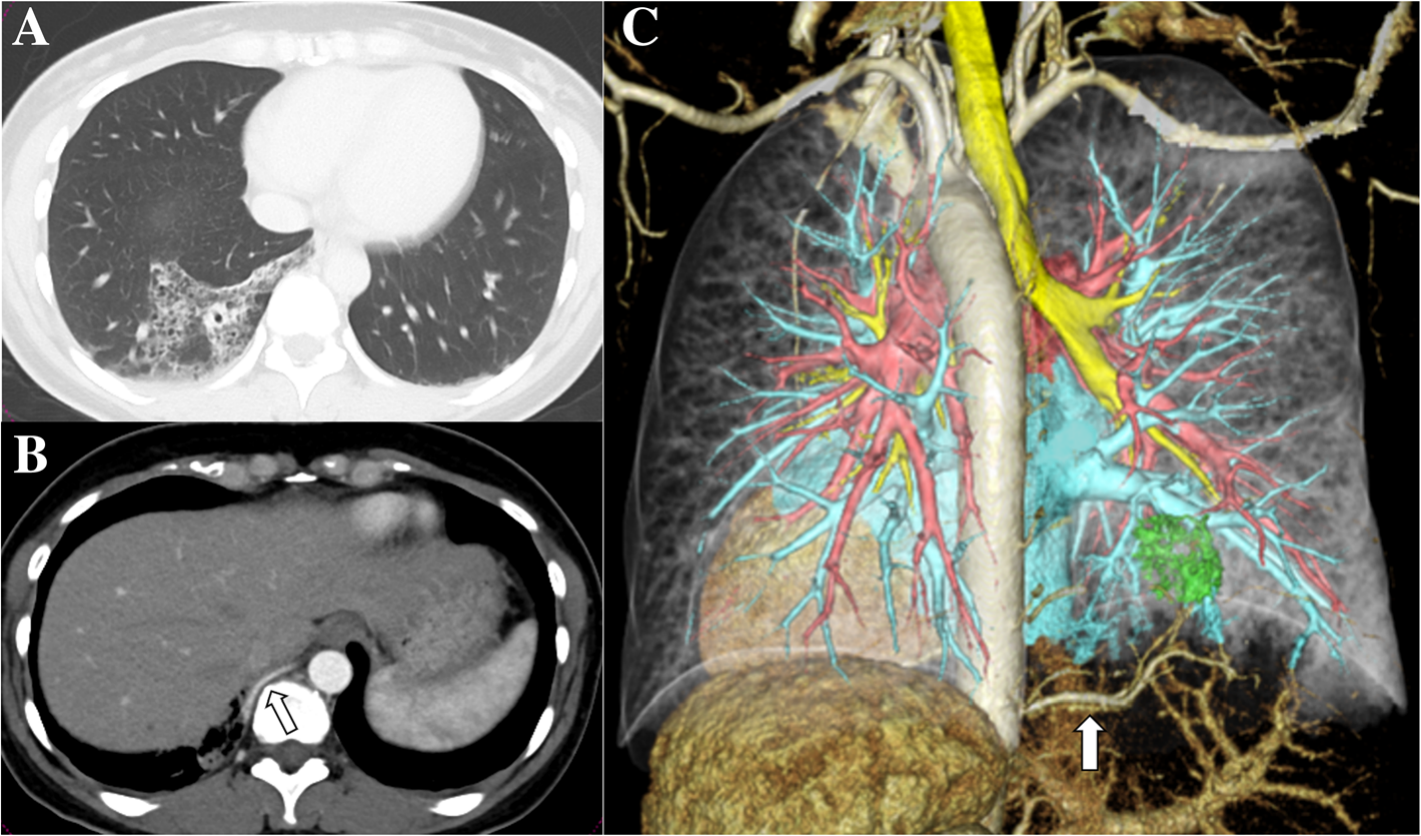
a Chest computed tomography showing multicystic changes in the lower lobe of the right lung. b Enhanced computed tomography showing the right aberrant artery arising from the descending aorta (*arrow*). c Three-dimensional computed tomography showing the right aberrant artery arising from the descending aorta (*arrow*)
